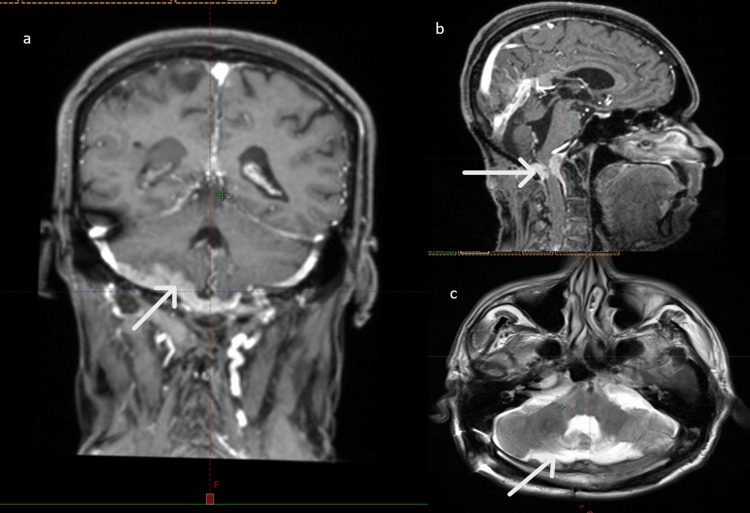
A relatively well-defined extra-axial lesion in the posterior cranial fossa on the right anterior aspect, appearing heterogeneously isointense on T2/FLAIR and hypointense on T1. The lesion shows heterogeneous post-contrast enhancement with extension into the superior aspect of the spinal canal, causing spinal cord compression. A skip lesion is also noted inferiorly within the spinal cord. The lesion appears as hyperdense areas in sagittal (B), coronal (A), and axial (C) images. FLAIR: fluid-attenuated inversion recovery
